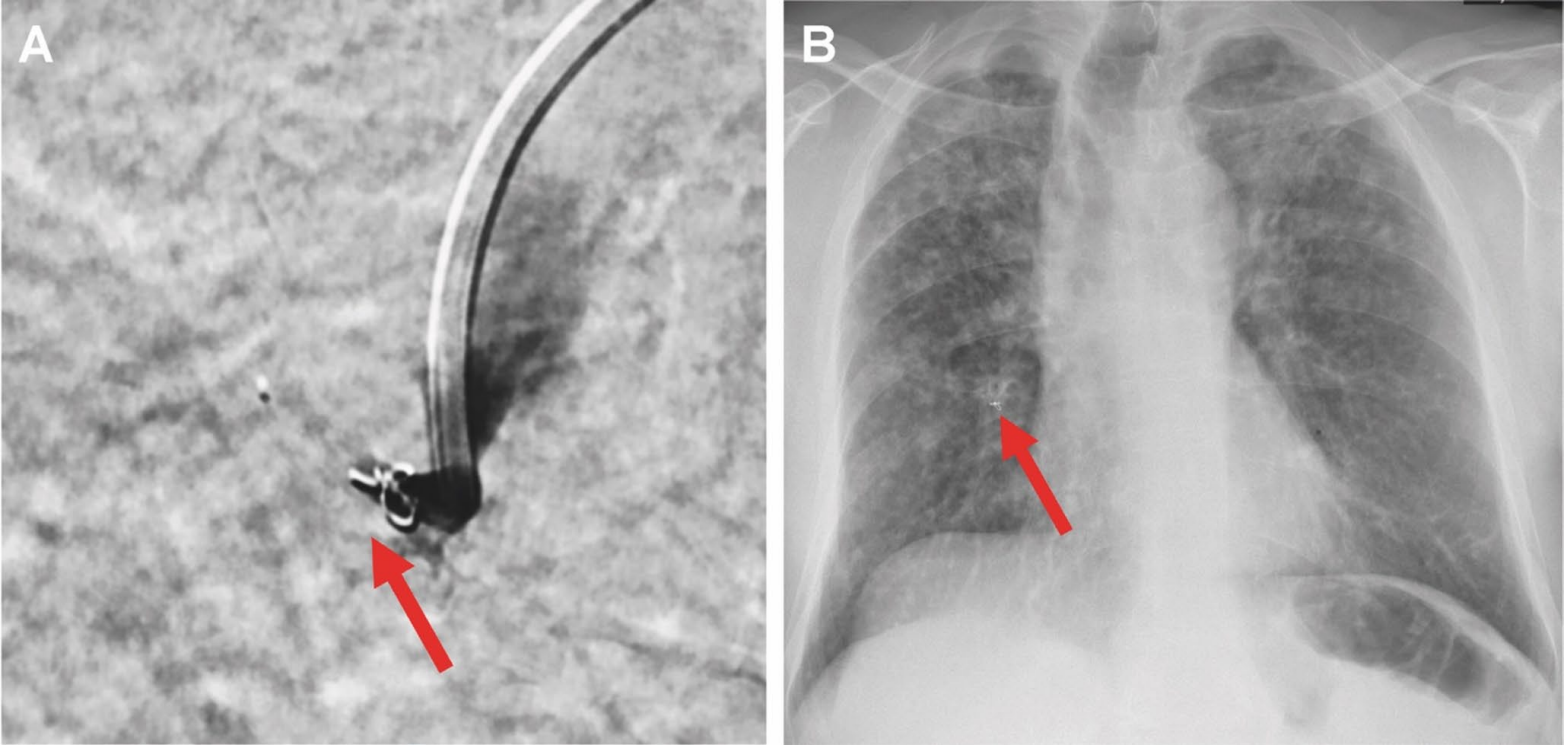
A Fluoroscopic image at the end of the intervention showing successful embolization of a Rasmussen aneurysm. A microvascular plug and a coil (red arrow) were deployed via a microcatheter into the targeted branch of the right pulmonary artery. Following plug and coil placement, selective contrast injection demonstrates complete occlusion of the aneurysmal vessel without residual filling — confirming the technical success of the embolization. B Post-interventional chest X-ray showing largely unchanged bilateral pulmonary opacities consistent with known cavities and infiltrates in the context of miliary tuberculosis. Newly visible foreign material (red arrow) is present in the right mid-lung field following embolization of a branch of the right pulmonary artery. No other significant interval changes are observed
